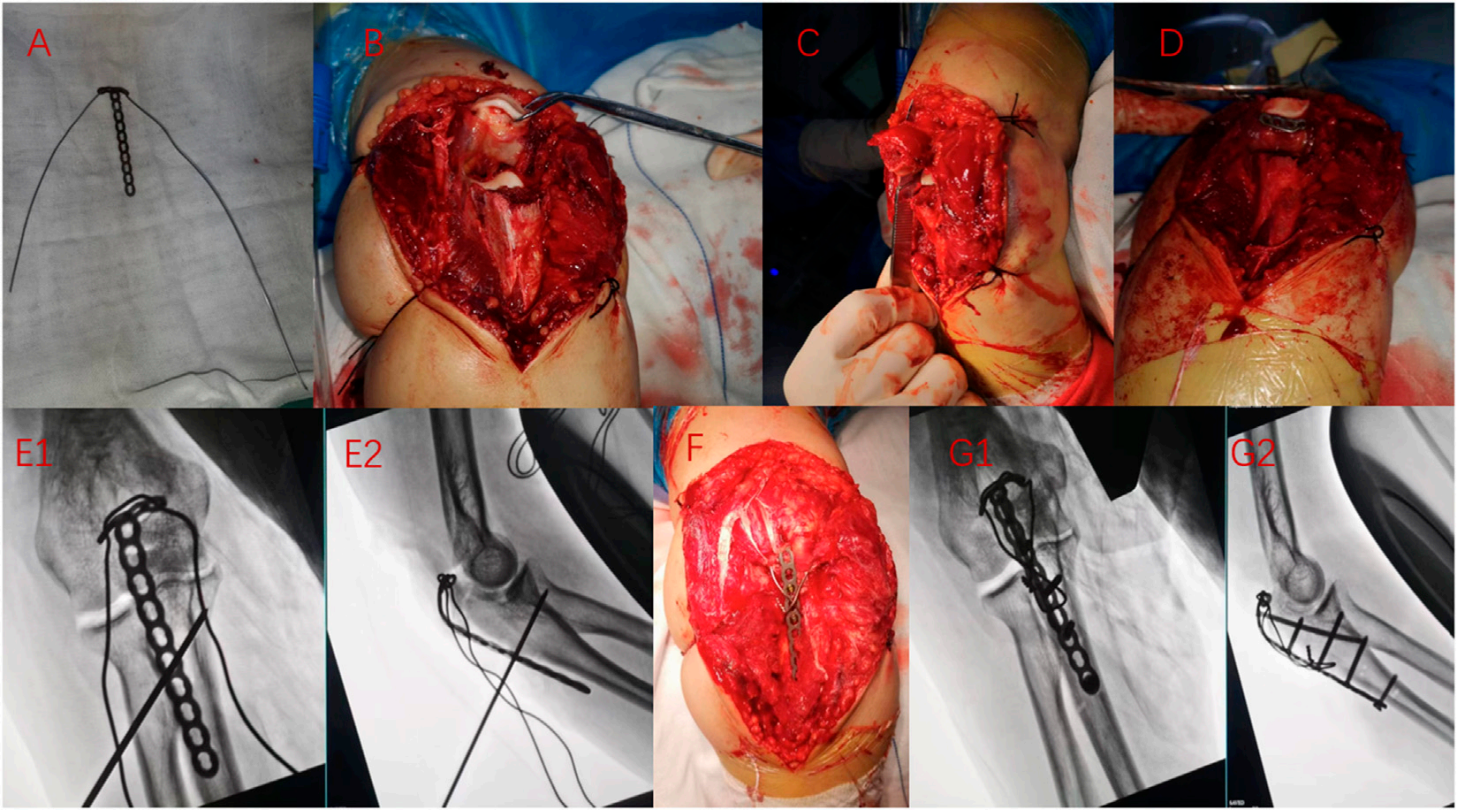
Intraoperative situation of anchor loop plate in treating olecranon fracture. (A) Bending micro steel plate, connecting steel wire; (B, C) Expose the fracture position, cut a small hole near the olecranon insertion of the triceps tendon; (D) Pierce the steel plate through a small hole from the inside out so that the short arm is suspended at the proximal end of the olecranon; (E1,E2) Drag the fracture block reduction, Kirschner wire temporary fixation; (F) Fix screw, binding wire; (G1,G2) Finally, intraoperative X-ray examination.
